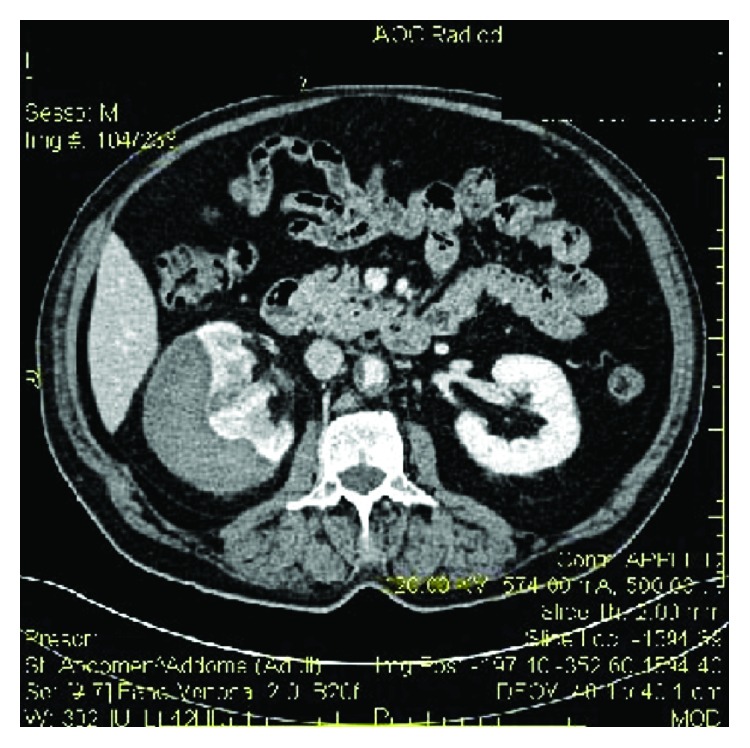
CT aspect of the hematoma.
